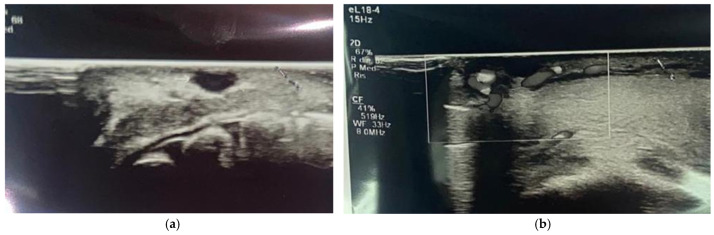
(a,b) Extracts from the labial ultrasound. Note the absence of vascularization or noble structures around the neoformation.
